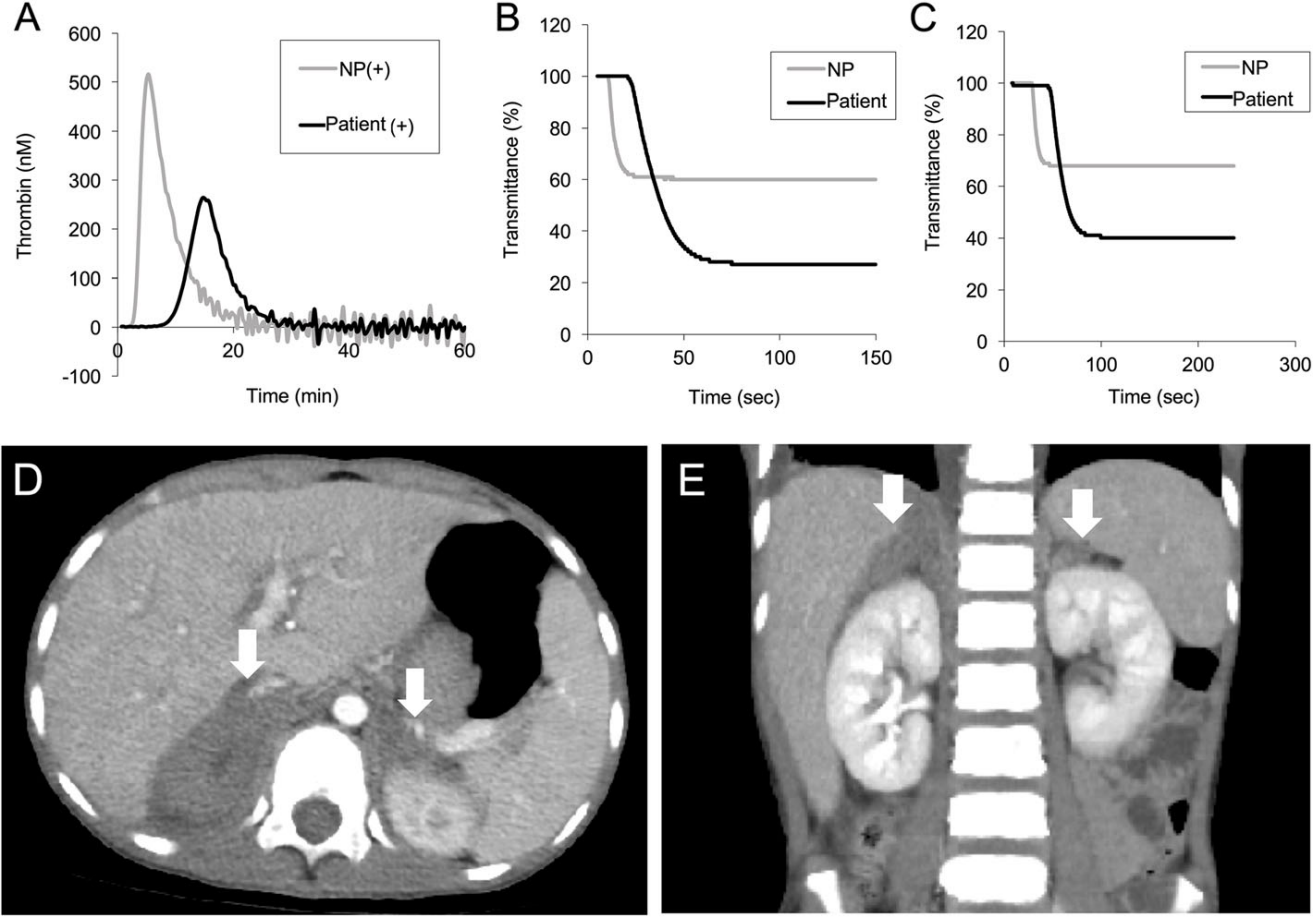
a Thrombin generation assay which monitors one-step before fibrin formation. In contrast to a healthy control, the lag time in lupus anticoagulant (LA)-positive serum was delayed. The peak thrombin level in the patient was lower than in the control. b and c Clot waveform analyses were evaluated by fibrin formation. The clot times in LA-positive serum were significantly prolonged compared to a healthy control. b, prothrombin time; c, activated partial thromboplastin time. d and e Contrast abdominal computed tomograms show nodular lesions in the enlarged adrenal glands bilaterally, indicating adrenal haemorrhage (arrows). d, Axial; e, Coronal
